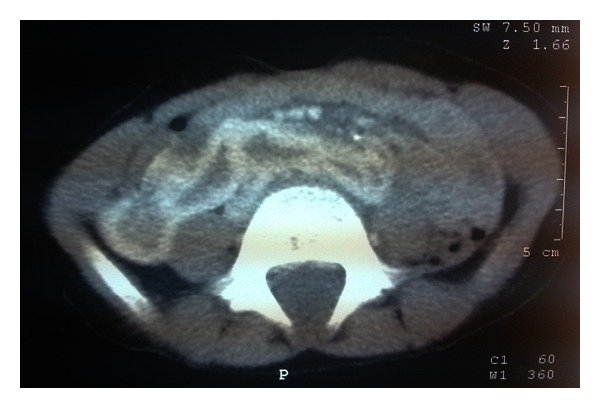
The presence of horseshoe kidney in abdominal ultrasonography.
